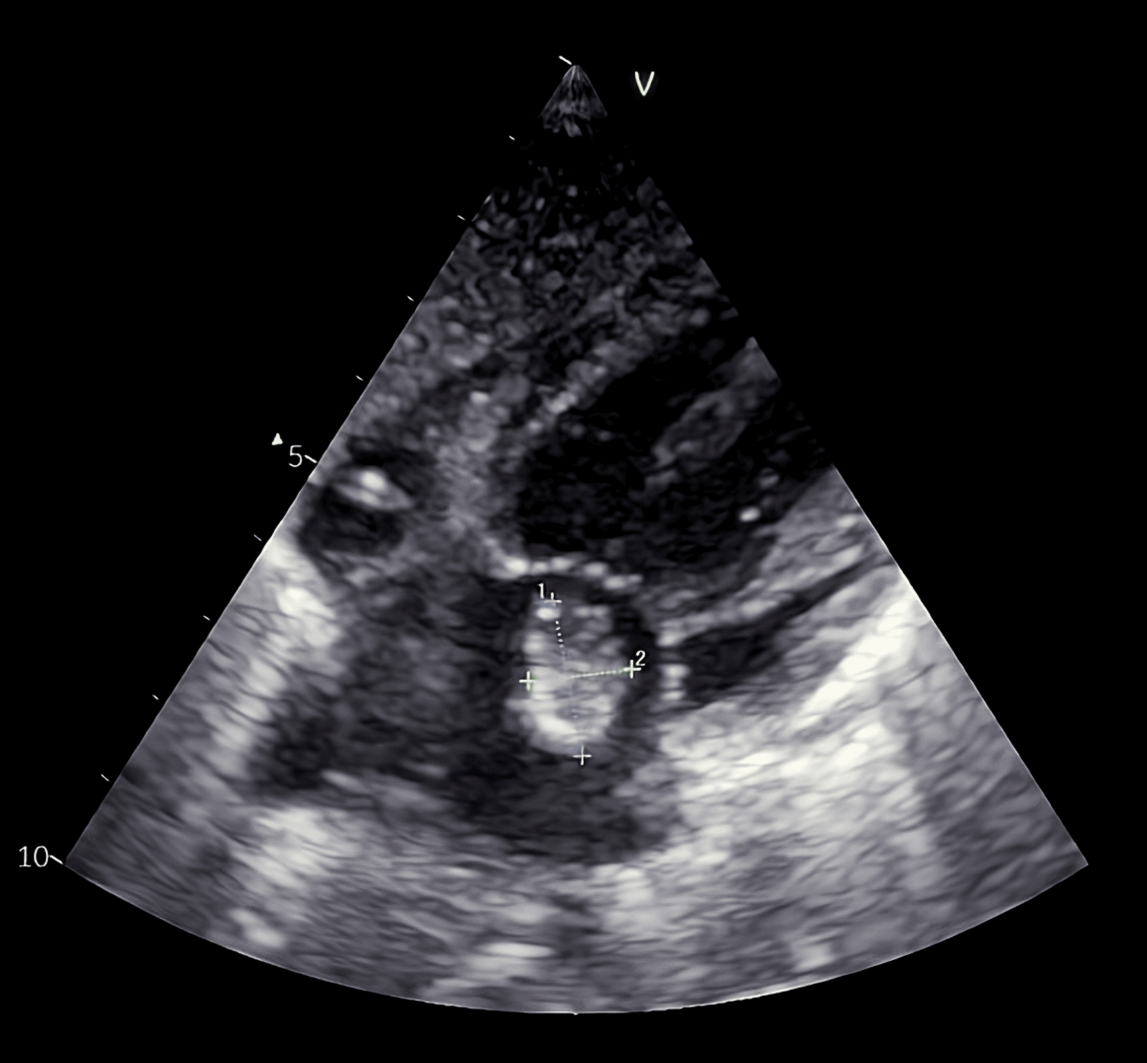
Subcostal view of the transthoracic echocardiography v: left
